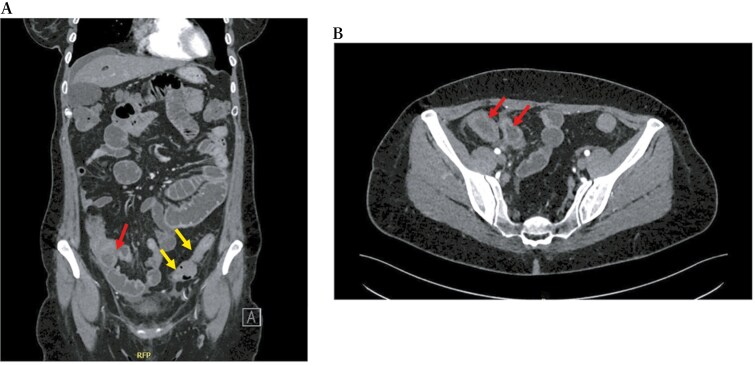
Computed tomography scan, in coronal (A) and transversal (B) sections, shows both a thickening of the last ileal loop for 5 cm in length with enhancement in the arterial phase (red arrows) and a concentric parietal thickening of the sigmoid colon (yellow arrows).
